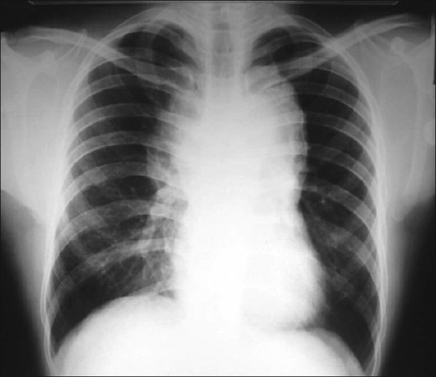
Plain chest X-ray shows large mediastinal mass
